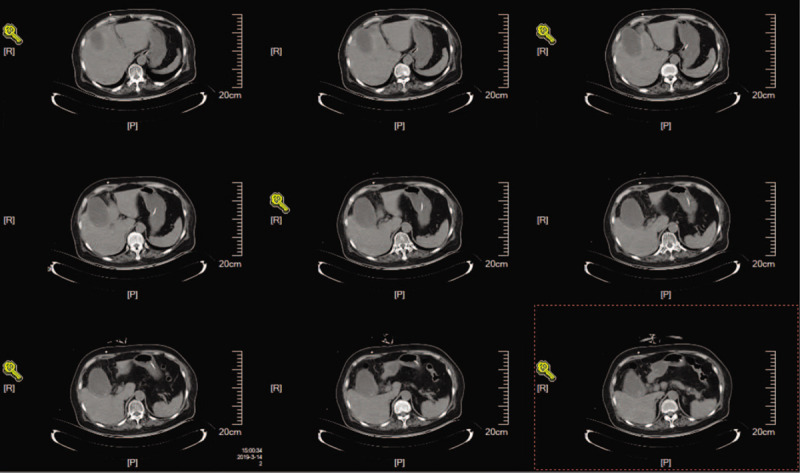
Preoperative abdominal computed tomography scan obtained during the second admission showed a swollen and enlarged gallbladder and flatulence.
